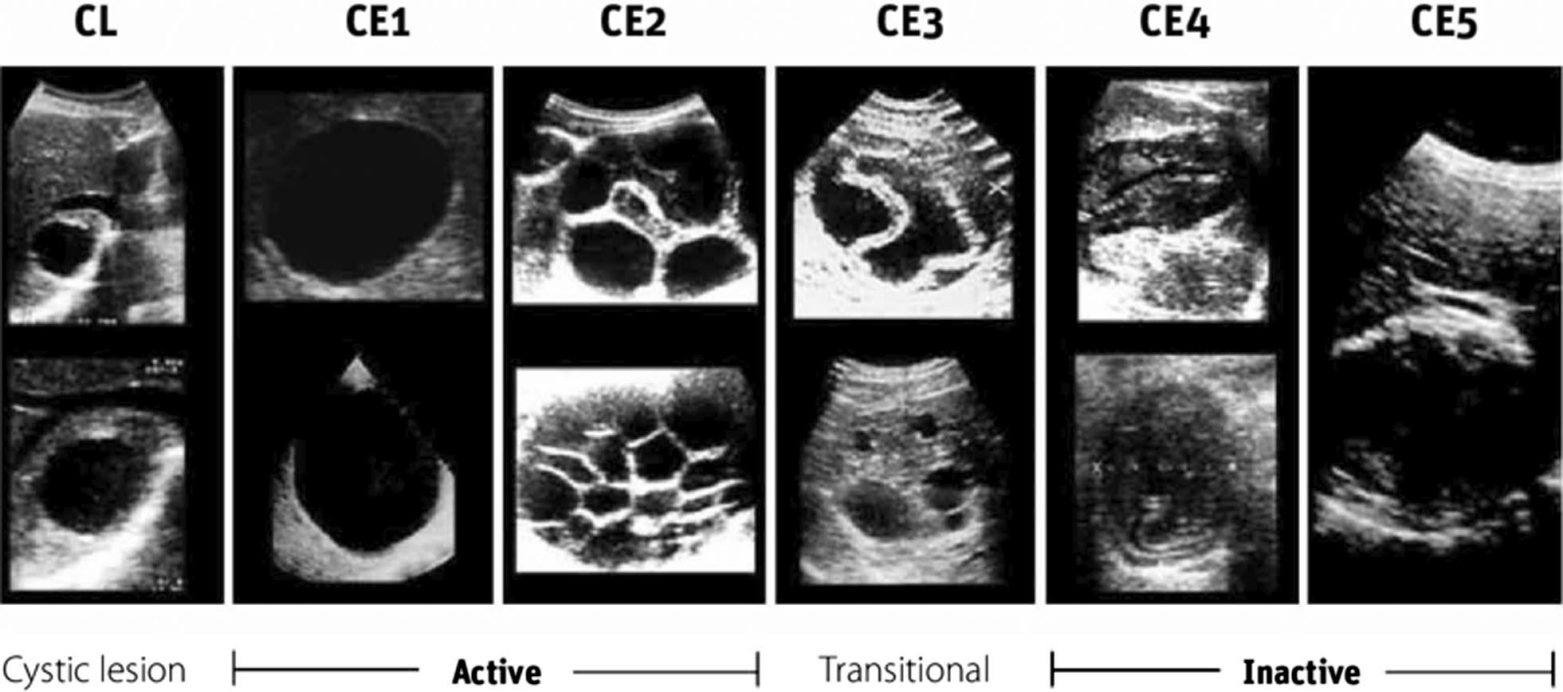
WHO-IWGE ultrasound standardized classification of echinococcal hepatic cysts © World Health Organization
